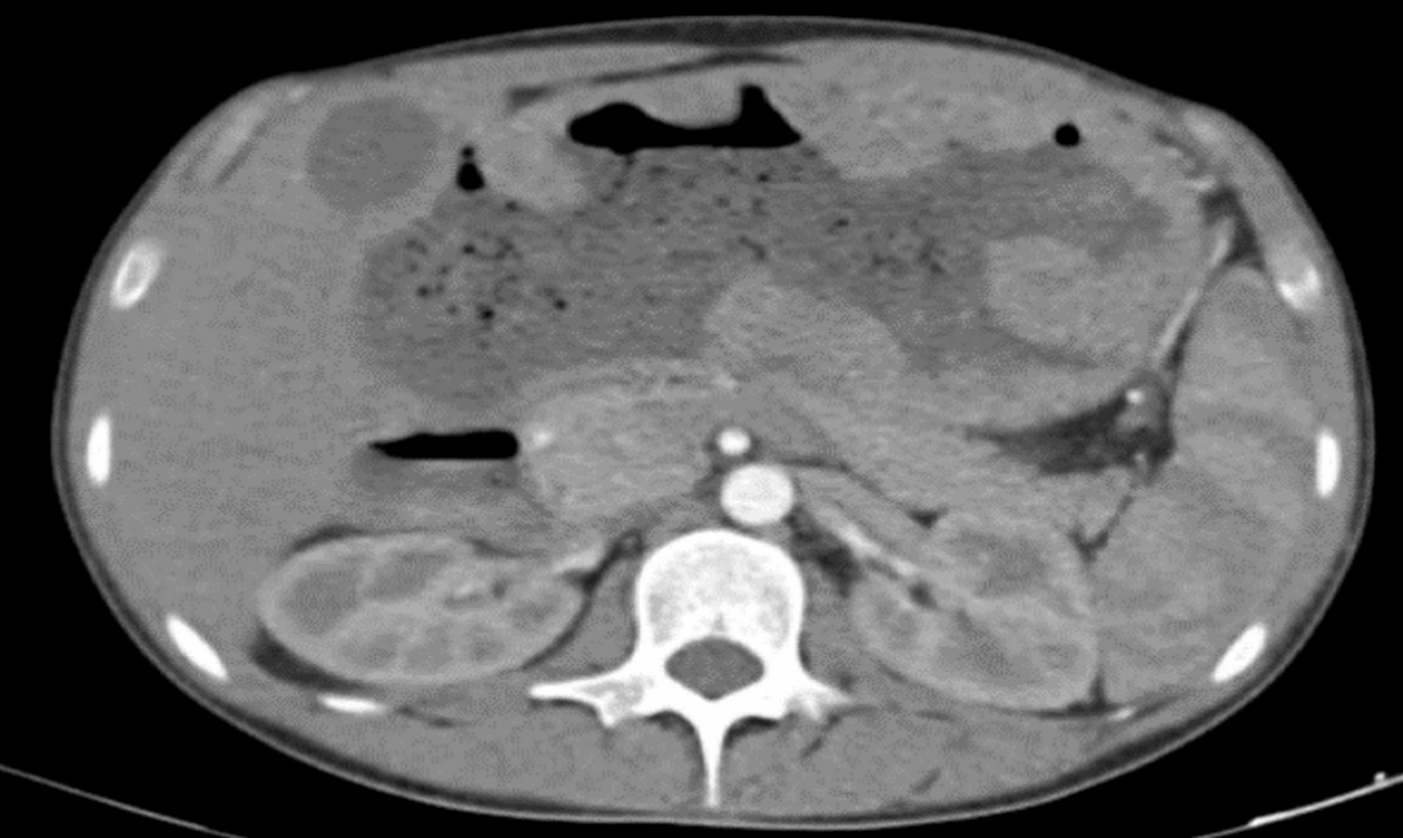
Axial contrast-enhanced CT images showing marked hypertrophy of the gastric folds, predominantly involving the fundus and body of the stomach
